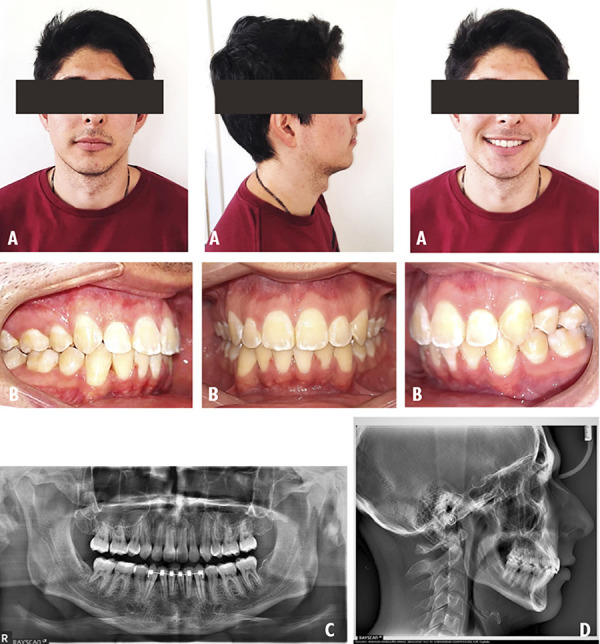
Case 2. Post-treatment records. A: Extraoral photographs. B: Intraoral photographs. C: Panoramic radiograph. D: Cephalometric radiograph.
